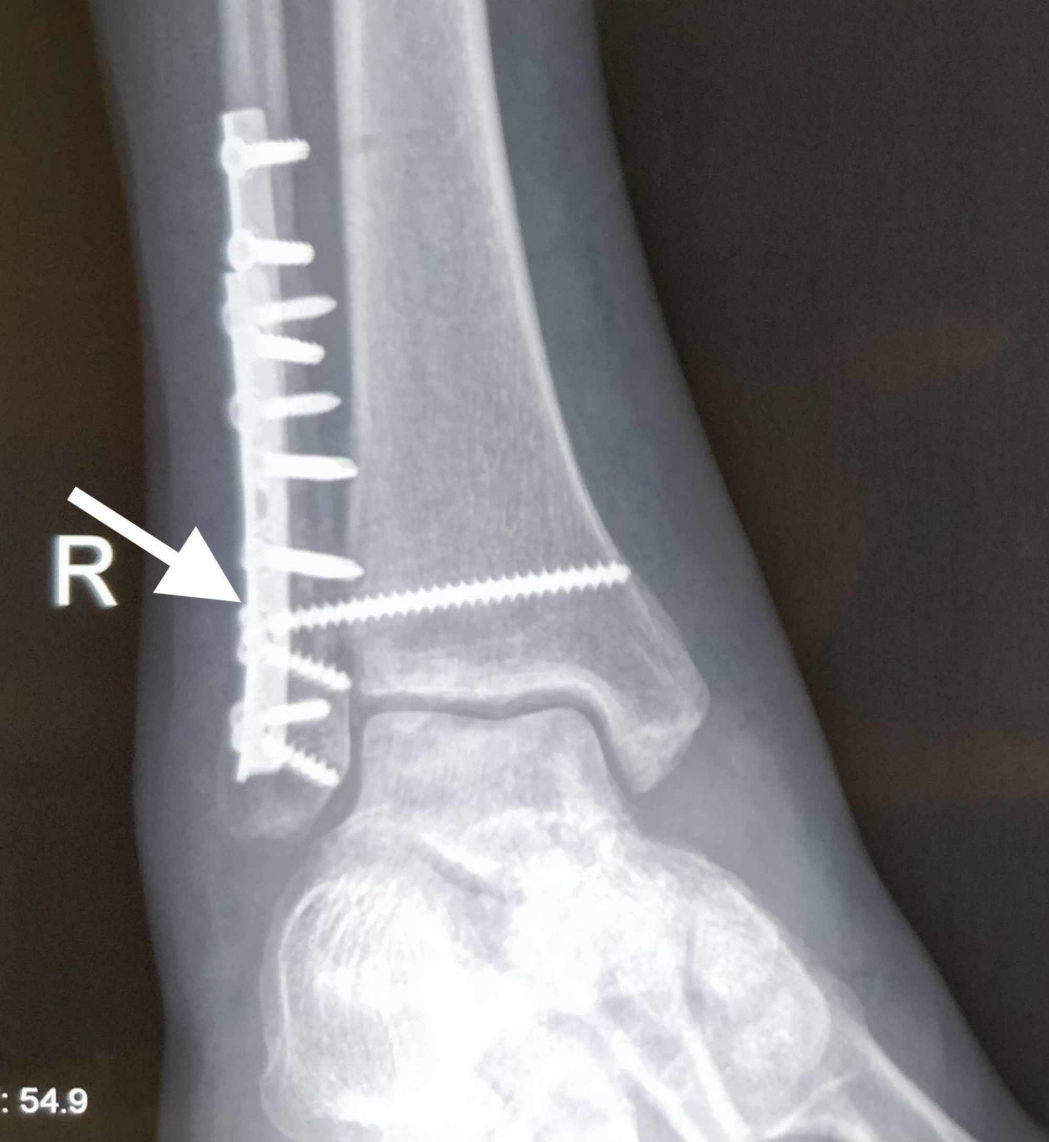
X-ray radiograph anteroposterior (AP) view showing the dual plating construct in an osteoporotic distal fibula fracture Note one plate applied on the lateral aspect of the fibula and the other on the posterior aspect of the fibula.
